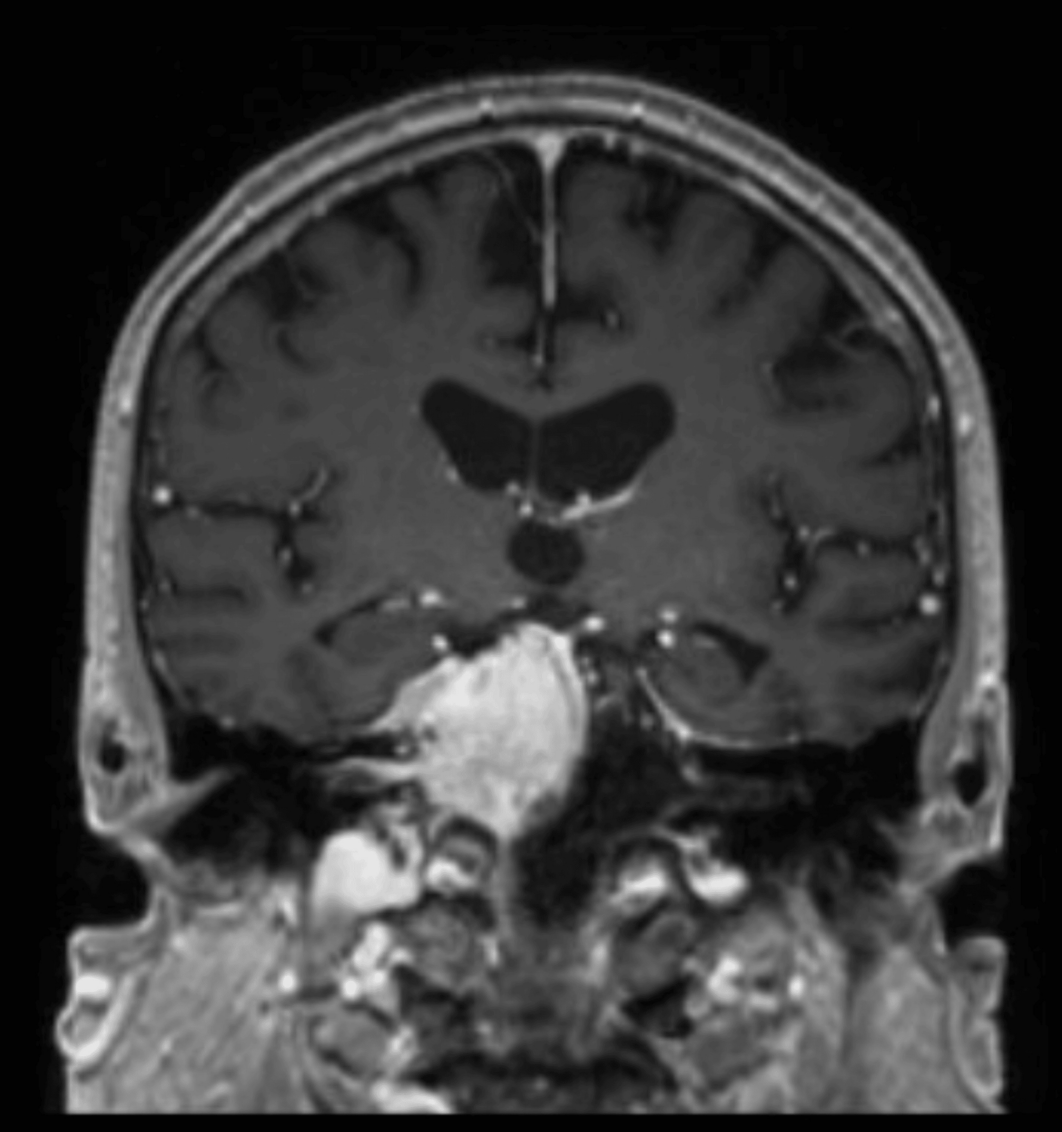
Pre-operative MRI with and without IV contrast Extra-axial mostly homogeneously enhancing mass extending into the sella and prepontine cistern with mass effect on the pons. Mass = 4.6 cm × 4.9 cm
